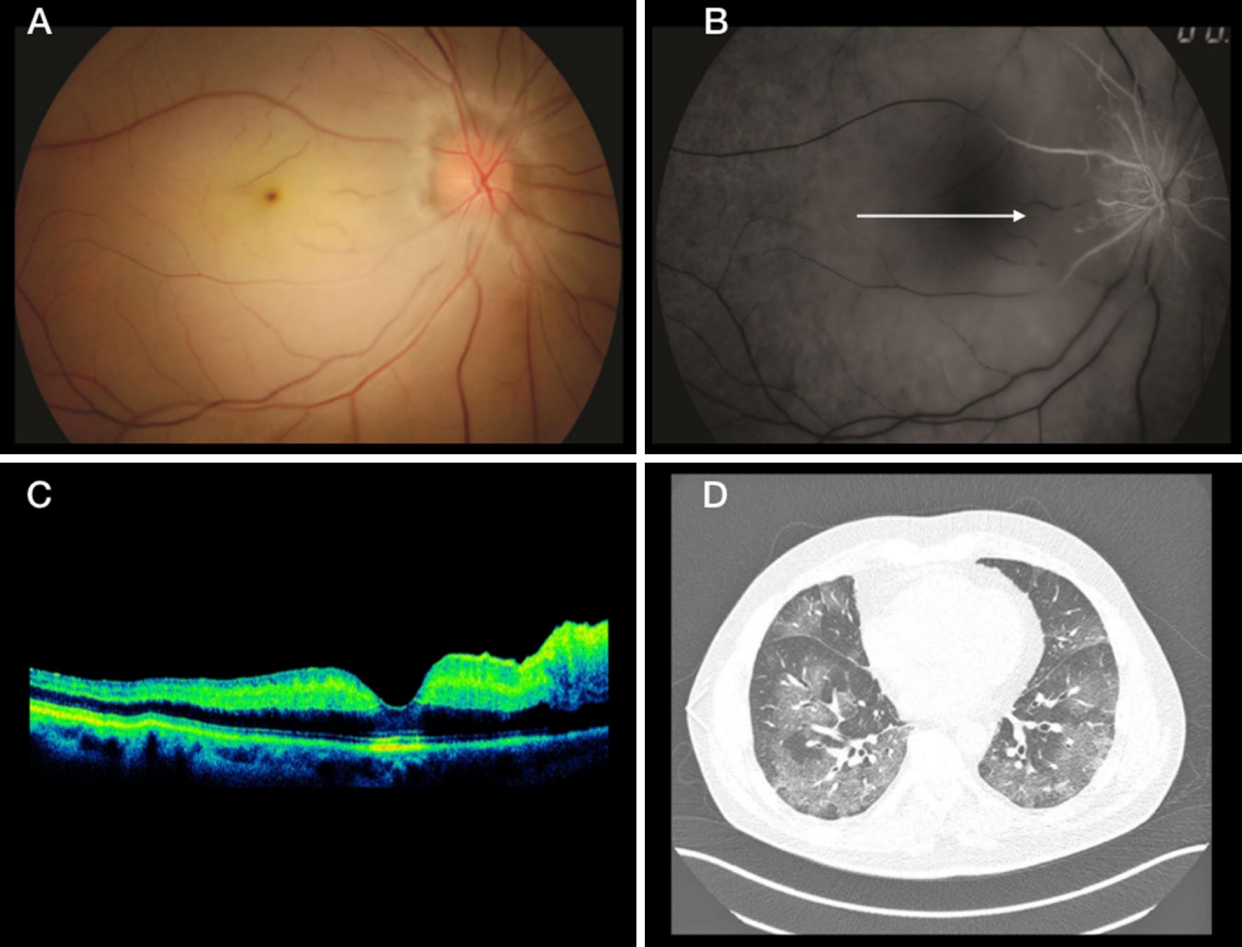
A Retinography of the right eye shows diffuse retinal paleness with a cherry-red spot in the macula. B Fluorescein angiography shows CRAO. C OCT image. D A chest nuclear magnetic resonance image. The arrow in B indicates occlusion of the cilioretinal artery
